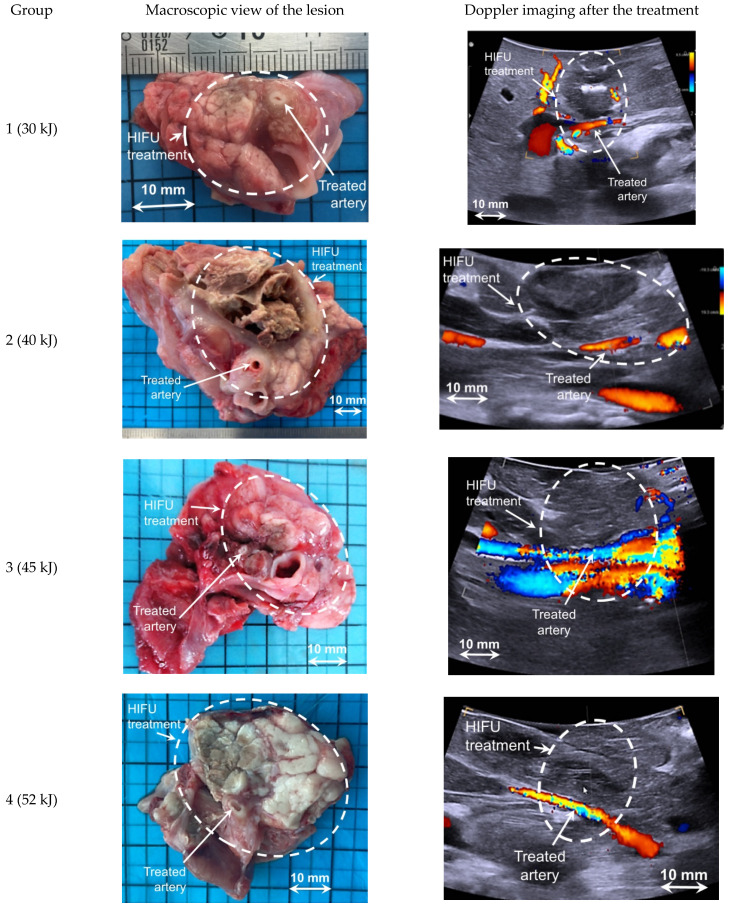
Typical examples for each group of HIFU ablations observed macroscopically and on ultrasound imaging 14 days after treatment.
